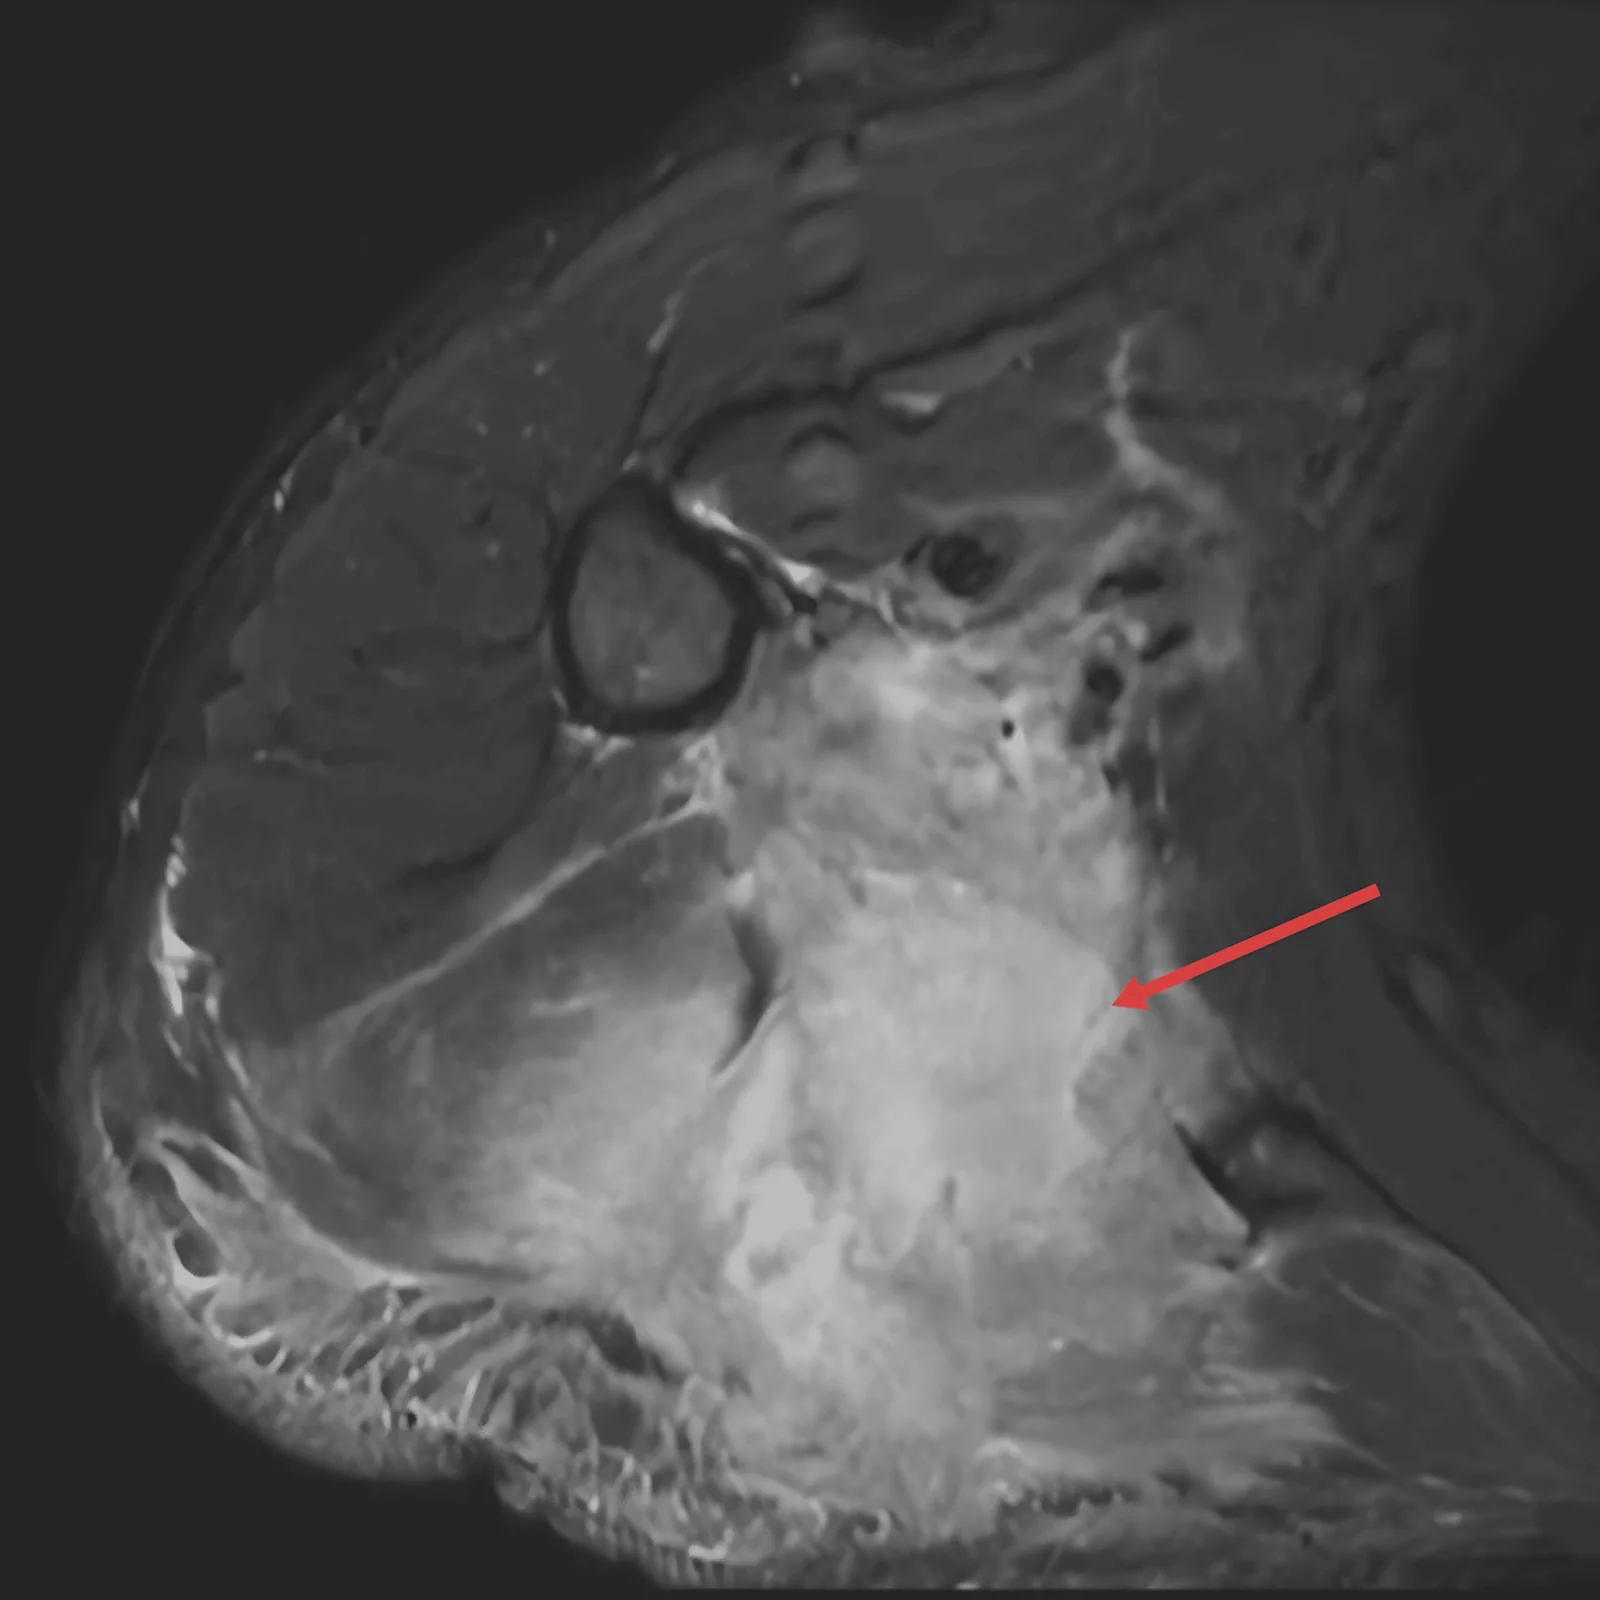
Preoperative MRI of the right shoulder demonstrating a complex fluid collection (arrow) centered on the right teres major region measuring 53 × 55 × 45 mm with extensive periscapular soft-tissue edema in the T2 axial view MRI: magnetic resonance imaging
